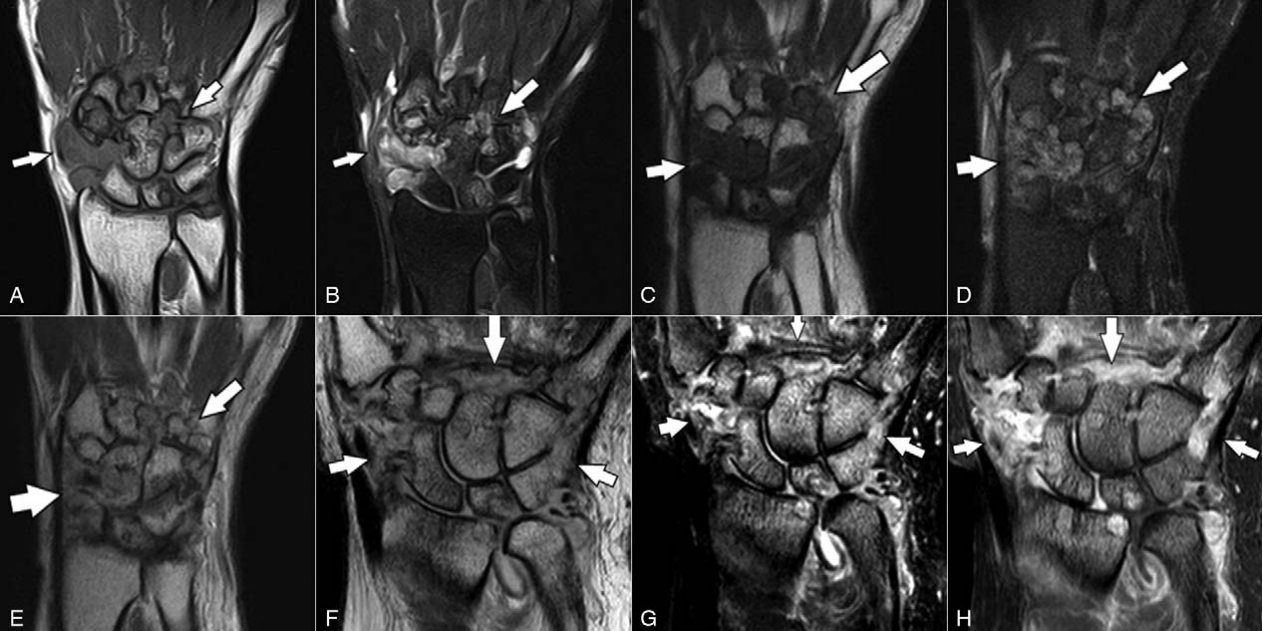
Magnetic resonance images of the 3 patients. In Case 1, multiple nodular, synovial, proliferative lesions, causing erosion of the carpal bones and metacarpal bases, revealed a low signal intensity on coronal T1-weighted imaging (A), and a heterogeneous high signal intensity on coronal fat-suppressed T2-weighted imaging (B) (arrows). In Case 2, multiple nodular, synovial, proliferative lesions, causing erosion of the carpal bones and metacarpal bases, showed a low signal intensity on coronal T1-weighted imaging (C), heterogeneous high signal intensity on coronal fat-suppressed T2-weighted imaging (D), and heterogeneous enhancement (E) (arrows). In Case 3, diffuse, synovial, proliferative lesions, causing erosion of the radius, carpal bones, and metacarpal bases demonstrated a heterogeneous high signal intensity on coronal intermediate (modified proton density-weighted) imaging (F) and fat-suppressed T2-weighted imaging (G), and heterogeneous enhancement (H) (arrows). The radiocarpal joint shows cartilage loss and joint space narrowing, with distal radioulnar compartment effusion. Diffuse bone marrow edema is seen in the distal radius and ulna, carpal bones, and metacarpal bases.
